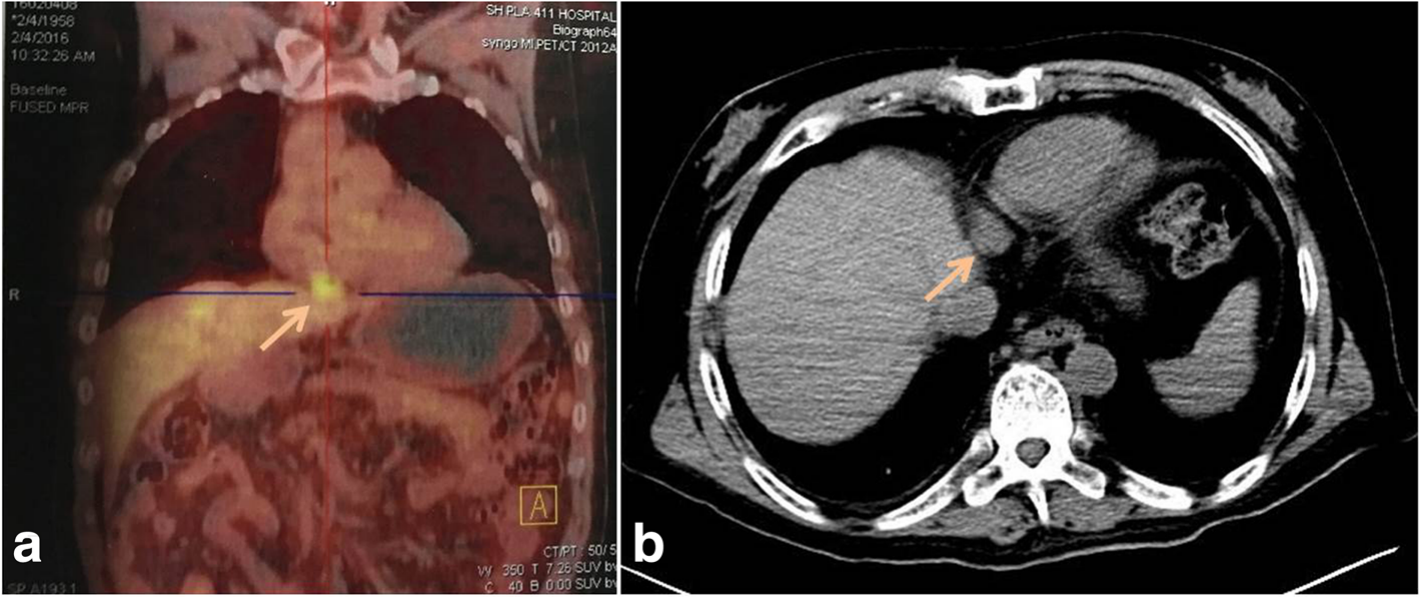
Preoperative images. a Follow-up positron emission tomography/computed tomography image at the local hospital; the *arrow* indicate the cardiophrenic angle lymph node metastasis. b Computed tomography image at our hospital; the size of the node is about 2.3 × 1.7 × 1.8 cm (*arrow*)
